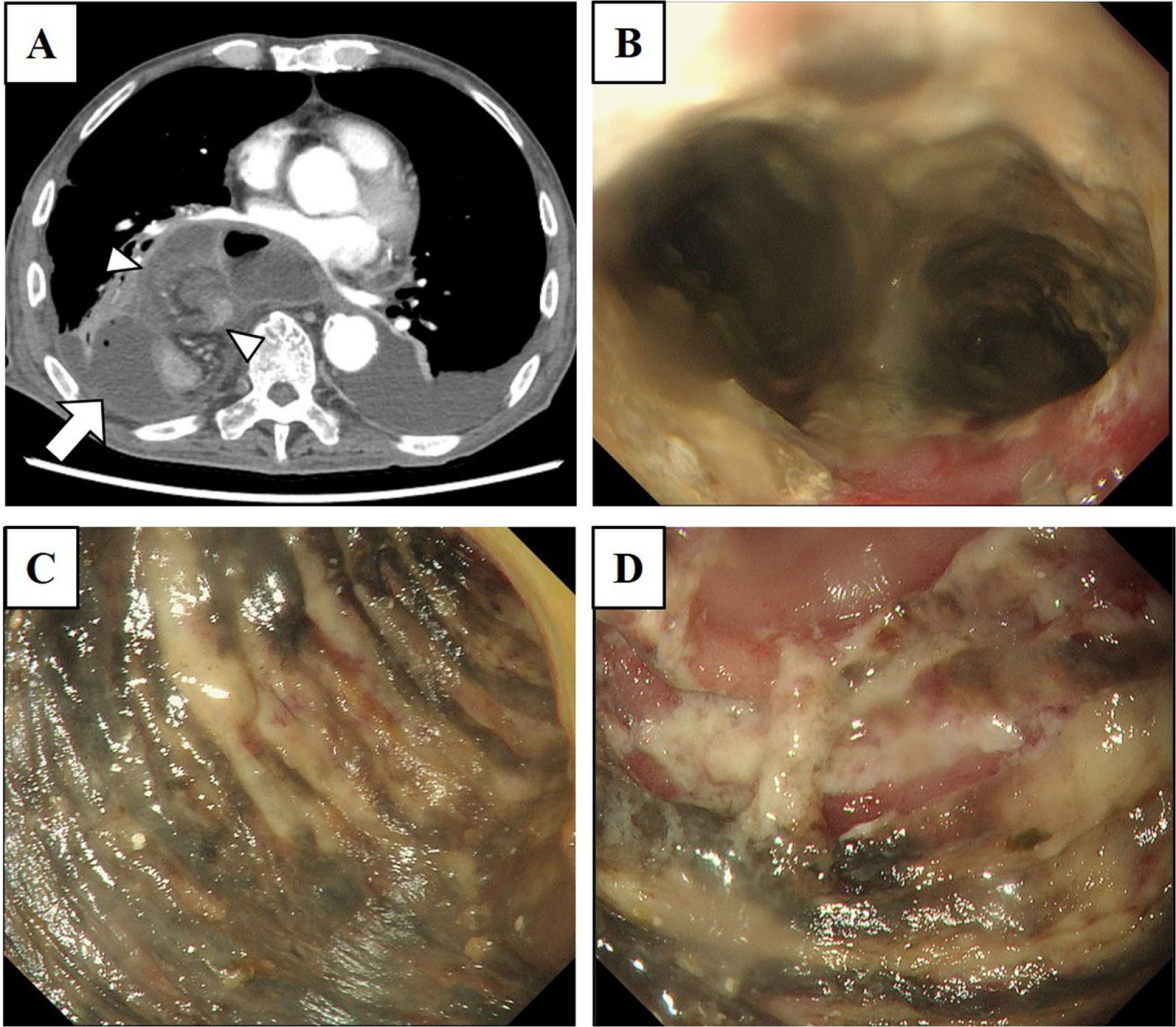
Imaging findings at the onset of nonocclusive mesenteric ischemia in the reconstructed jejunum. A Contrast-enhanced computed tomography showed diffuse wall thickening of the reconstructed jejunum elevated in the thoracic cavity with poor contrast enhancement (white arrowhead). The arterial and venous flow in mesentery of the reconstructed jejunum were maintained. A fluid correction with tiny free air was observed in the dorsal side of right thoracic cavity (white arrow). B In esophagogastroduodenoscopy, an apparent mucosal necrotic finding was observed at the most oral side of the reconstructed jejunum. C Segmental mucosal necrotic change was observed in the reconstructed jejunum elevated in thoracic cavity. D No necrotic finding was observed in the jejunum in abdominal cavity
